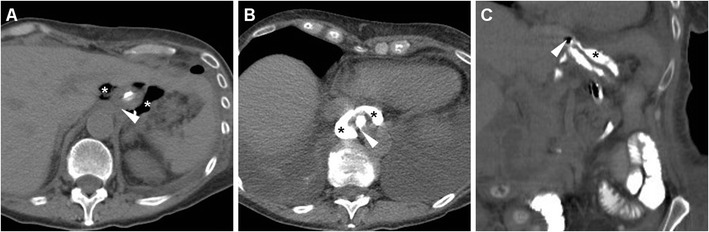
In a 76-year-old woman with T4 N2 gastric cancer, three days after total gastrectomy unenhanced CT (A) showed minimal residual pneumoperitoneum and extensive air (*) surrounding the EJS (arrowheads). Repeated unenhanced CT with oral contrast administration (B, C) showed patent EJS (arrowheads) and confirmed circumferential anastomotic leakage (*), which was initially managed conservatively but ultimately required reintervention with redo anastomosis six days later because of failed clinical improvement and progressive anemization
